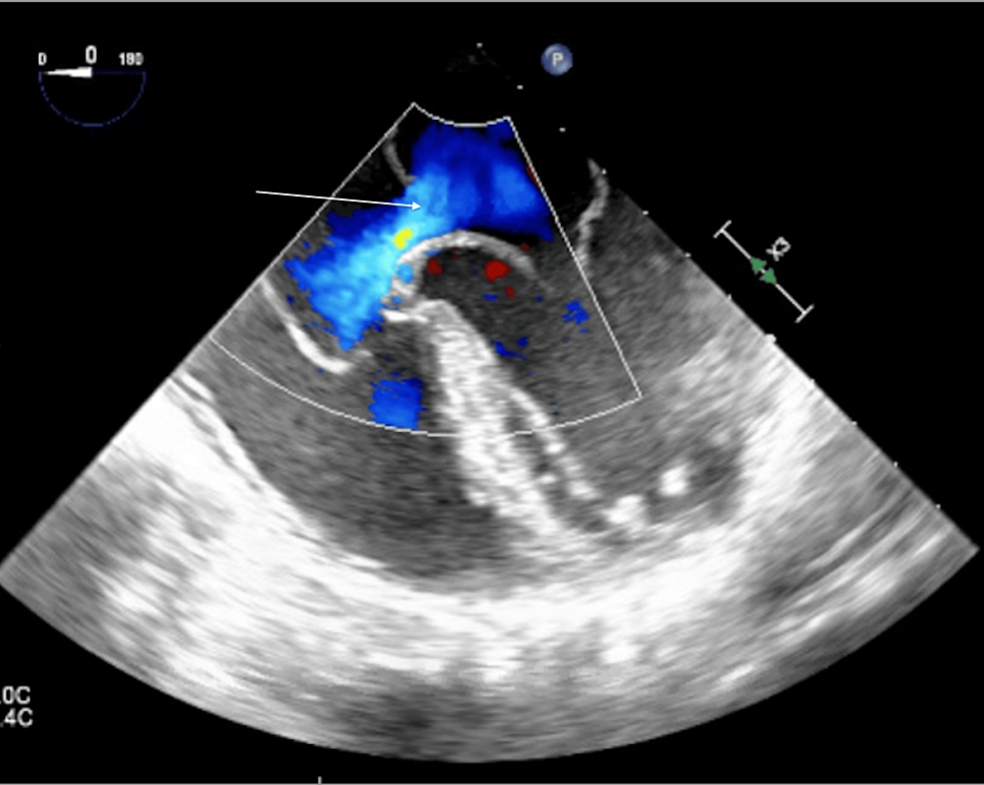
Intraoperative TEE showing the atrial septal defect TEE, transesophageal echocardiography
